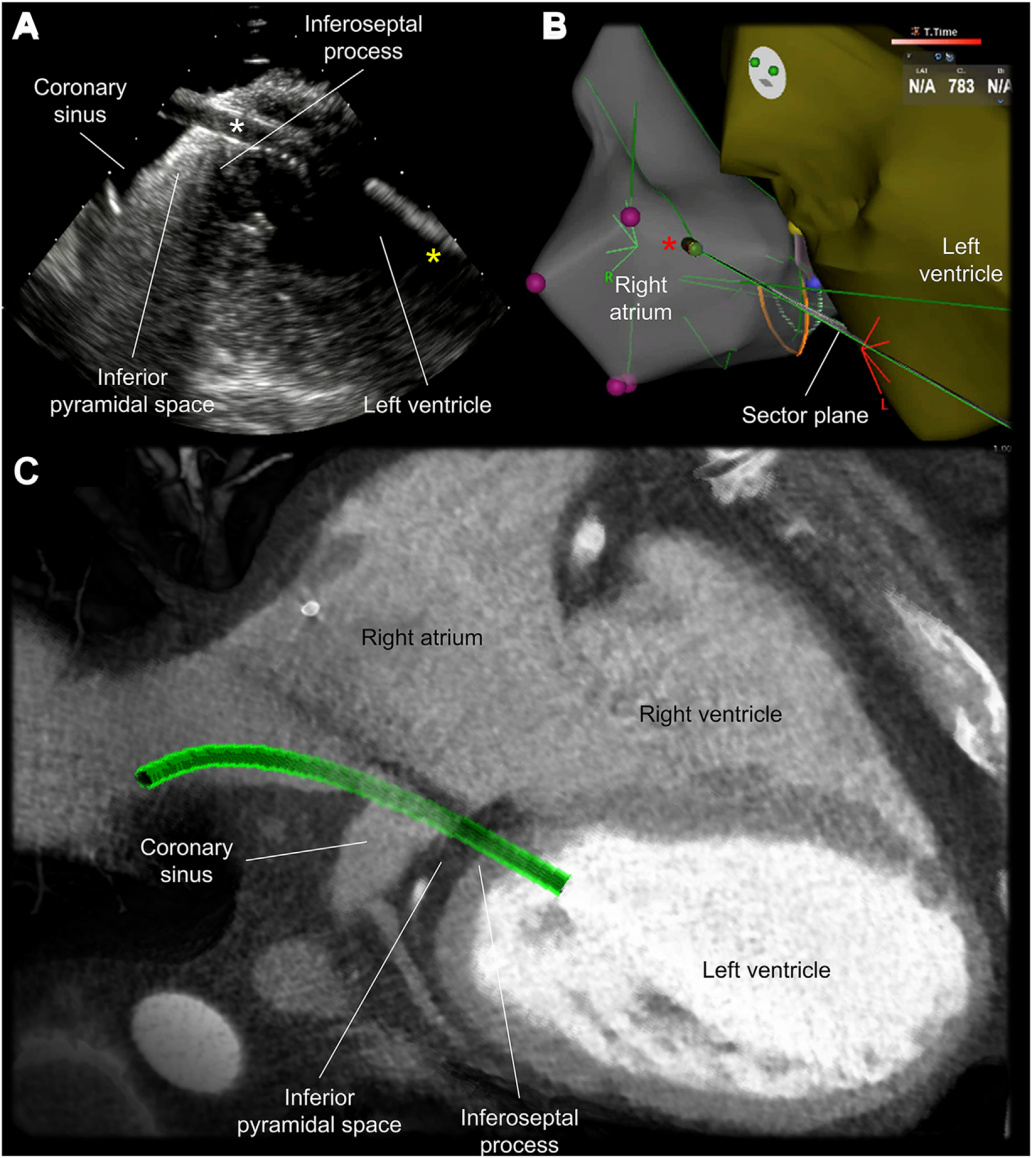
Intracardiac echocardiography. A, B: The procedure was performed with real-time assistance of an intracardiac echocardiography. The *white and yellow asterisks* indicate a sheath passing from the right atrium to left ventricle and a catheter placed in the left ventricle, respectively. The *red asterisk* denotes the intracardiac echocardiography probe. C: replicates the sectional plane of the intracardiac echocardiography (A) with a virtual sheath (*green*).
